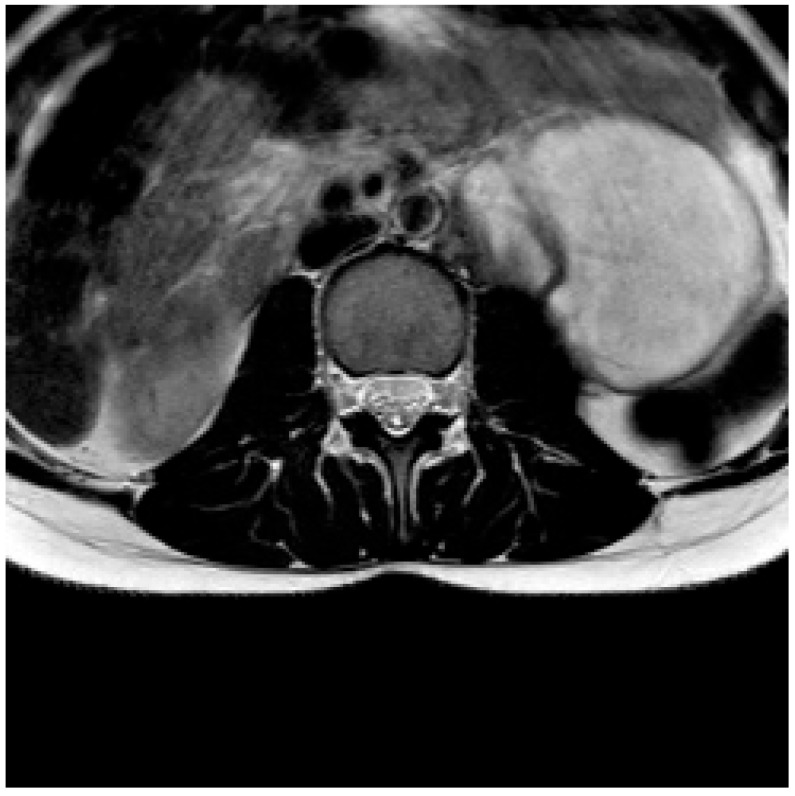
The axial T2-weighted sequence shows a cystic retroperitoneal paravertebral left mass as an occasional finding.
